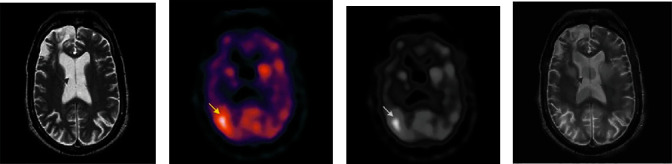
(a) Input image-1 (MRI-brain tumor. (b) Input image-2 (SPECT-brain tumor. (c) Converted grey scale image of input image-2. (d) Fused image (MRI-SPECT).
